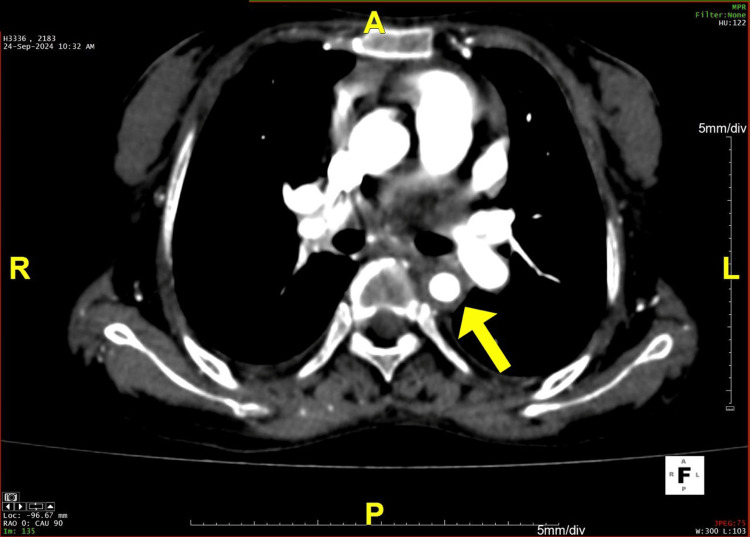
An axial section of the CT angiogram shows narrowing of the descending thoracic aorta CT: computed tomography
